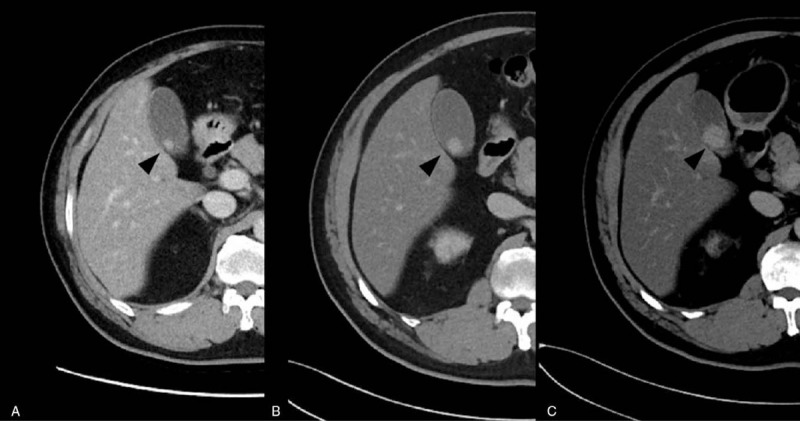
Computed tomography shows growth of the gallbladder polyp (arrowhead). (A) 1 yr after the nephrectomy. (B) 34 mo after the nephrectomy. (C) 38 mo after the nephrectomy.
